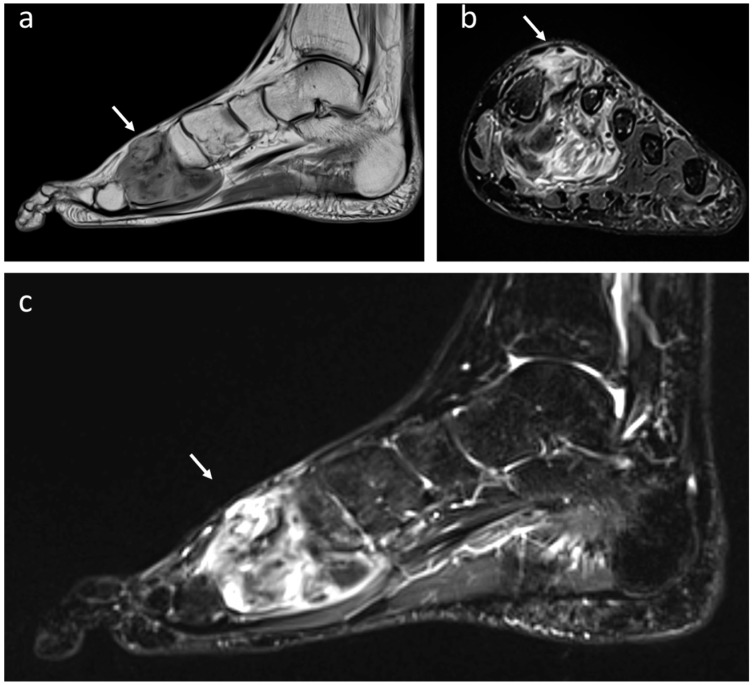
Synovial monophasic Sarcoma of deep foot soft tissues (white arrows) in a 49-year-old male patient: (a) T1-weighted MRI showing heterogeneous multiloculated mass isointense to muscle in sagittal projection while an increased signal intensity is shown at T2 STIR in coronal (b) and sagittal projection (c).
